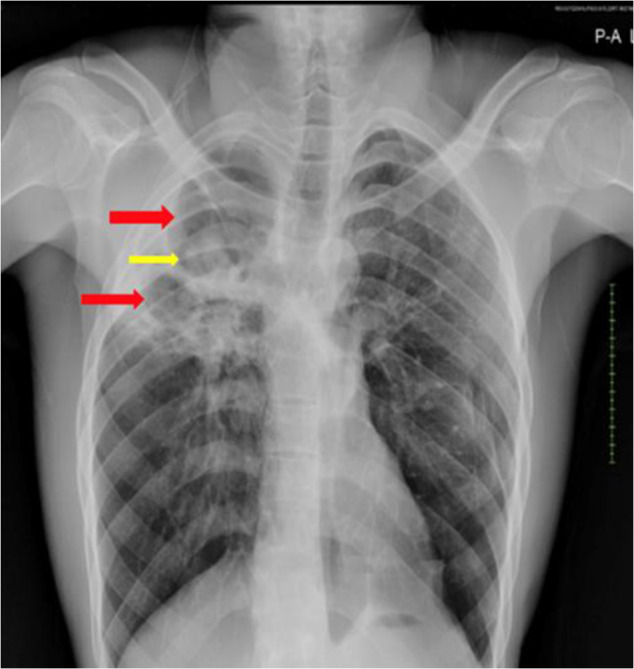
Patient's chest radiograph showing thin-walled right upper lung zone cavities (red arrows) with a soft tissue density lesion (probably aspergilloma) within the dependent portion of the superior cavity (yellow arrow). An air crescent sign (Monod's sign) is seen surrounding another aspergilloma in the smaller cavity close to the right hilum.
